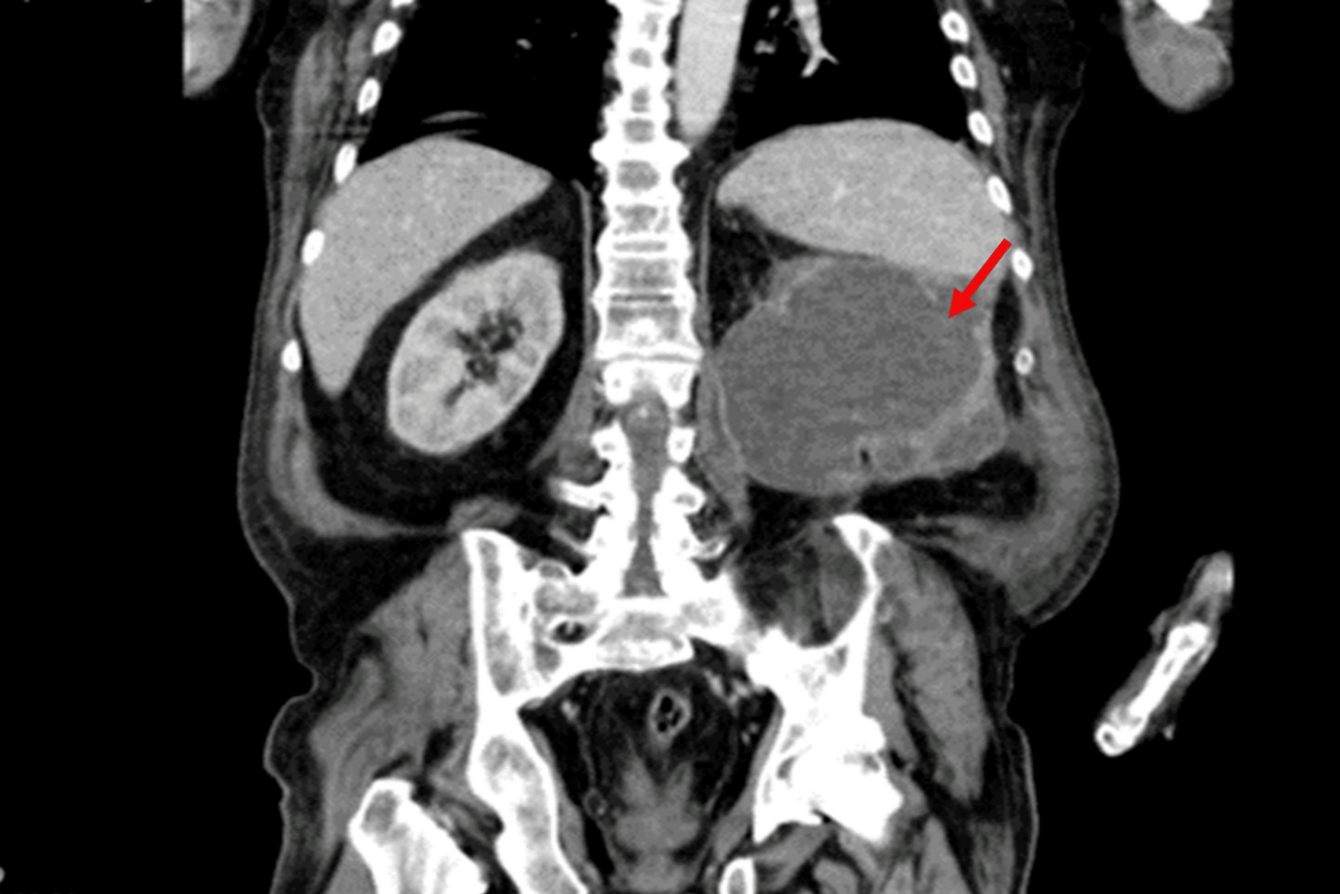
Abdominal CT scan at admission showing well-defined left cystic renal mass (13 cm) consistent with a cystic nephroma, associated with mild hydronephrosis and renal parenchymal atrophy
